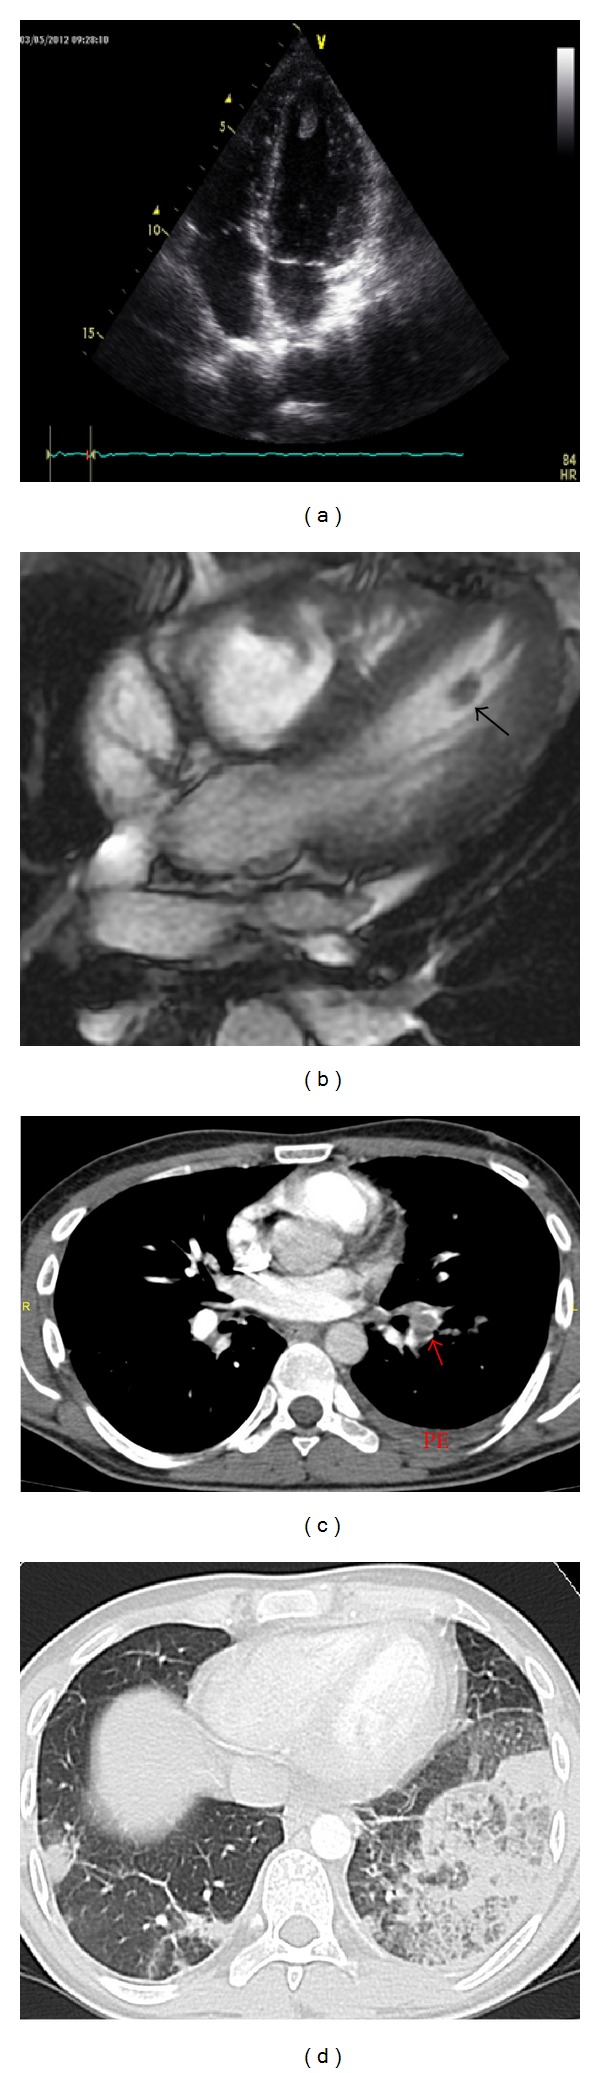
(a) Transthoracic echocardiographic apical four-chamber view shows a thrombus in the apex of the left ventricle. (b) Four-chamber-view MR image obtained with fast imaging steady-state free precession sequence shows pedunculated thrombus (arrow) attached to left ventricular apex with a thin stalk (cine images showed mobility of the thrombus throughout the cardiac cycle). (c) Pulmonary CT angiography shows acute embolus as a filling defect in the left lower lobe artery (arrow). Note the associated left pleural effusion (PE). (d) Lung window setting shows bilateral lower lobe infarcts, more prominent in the left lung.
